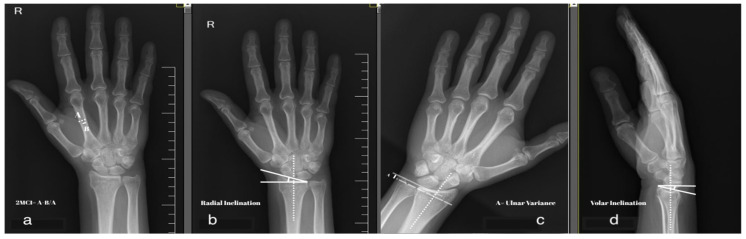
(a–d): Radiographic measurements.
